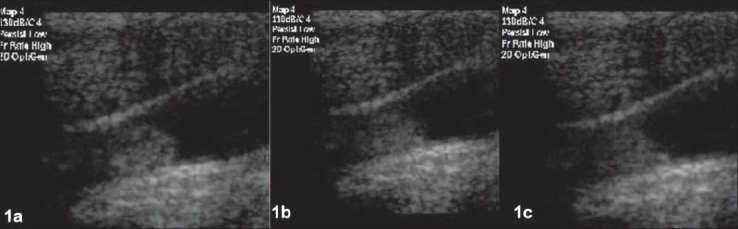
(a, b, c) B-mode carotid doppler (longitudinal) showing the mobile plaque in the proximal left ICA moving in sequence during systole
